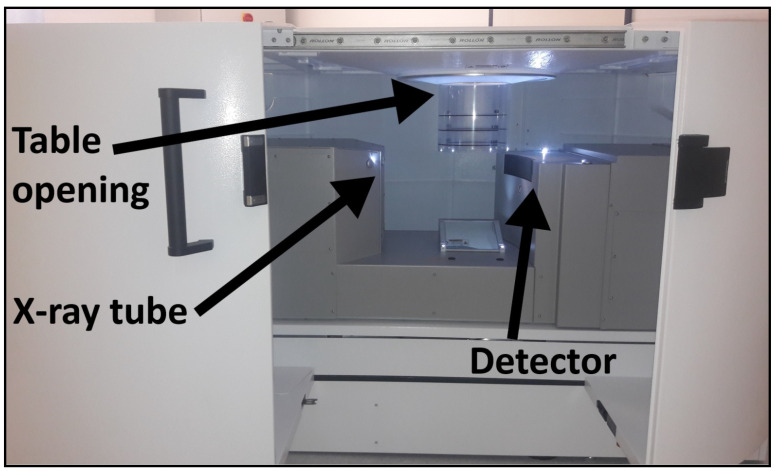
Design of the dedicated spiral breast CT. The patient lies on the patient table in prone position. The concave shape of the patient table around the table opening enables imaging of breast tissue up to the chest wall. The breast hangs freely through the table opening and is covered by a cylindric safety cover. The x-ray tube/detector unit rotates on a spiral path around the breast.
